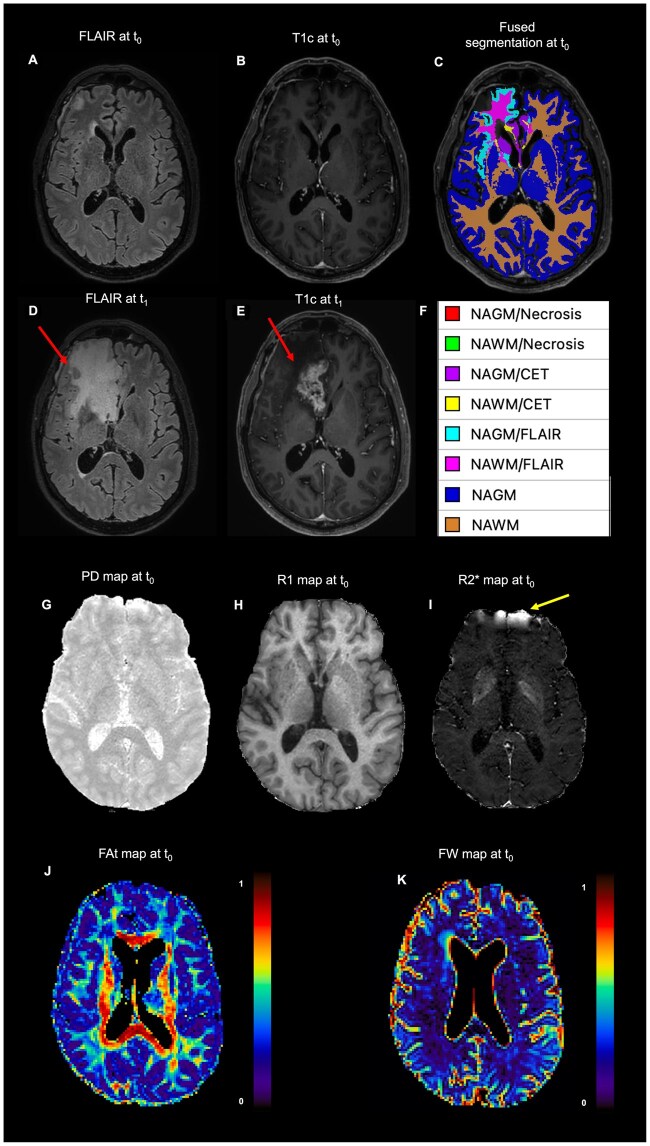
A 59-year-old male patient with glioblastoma WHO CNS grade 4: Routine scans for response assessment (*t*_0_) with no signs of tumor recurrence in axial FLAIRw sequence (A) and T1w post-contrast (T1c, B). Stable disease according to RANO 2.0. (D + E) The same patient 3 months later (t1). Extensive tumor recurrence with new FLAIR-hyperintensities, CET, and necrosis in the right frontal lobe (see arrows in D and E). Progressive disease according to RANO 2.0. (C) Fused segmentation of subregions shows normal-appearing tissue at *t*_0_ that will progress to tumor regions at (*t*_1_). Note that preexisting FLAIR-hyperintensities in the right frontal lobe and adjacent to the right lateral ventricle were manually excluded. (F) shows the different subregions. The violet label, for instance, depicts NAGM in the routine scan, which became CET in the follow-up scan. Quantitative maps at *t*_0_:(G) PD map, (H) R1 map, (I) R2* map. Note the susceptibility-artifacts close to the frontal sinuses in the R2* map ( arrow in I). DTI-derived metrics at t0: FAt map (J), FW map (K).
